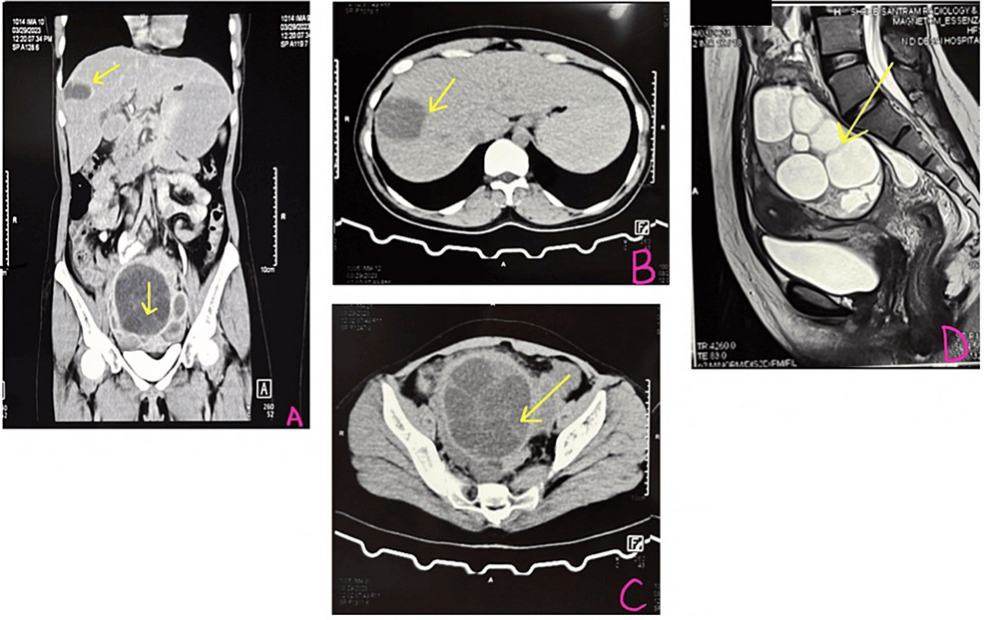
A: Coronal section on CT scan showing liver and pelvic hydatid cysts (yellow arrow); B & C: Axial section on CT scan showing liver and pelvic hydatid cysts, respectively (yellow arrow); D: Sagittal section on a CT scan showing a pelvic hydatid cyst abutting the bowel (yellow arrow) CT: computed tomography
